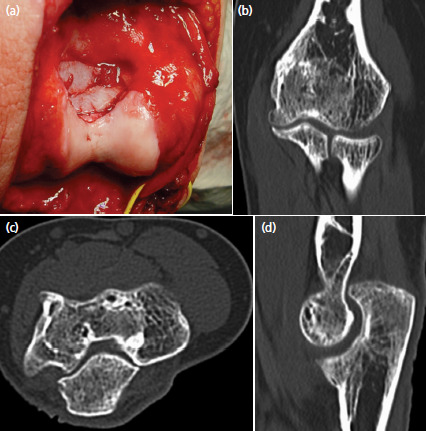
Intra- and post-operative images of the same elbow: (a) posterior view after autologous bone grafting. (b) Coronal, (c) axial and (d) sagittal CT-images made three years after tumour removal. The trochlea is anatomically shaped and filled with cancellous bone.
